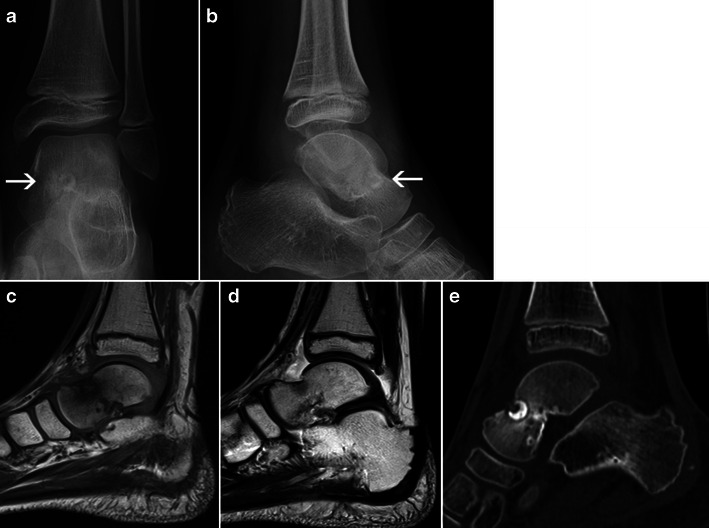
Osteoid osteoma of the left talar neck: Frontal (a) and lateral (b) X-rays of the left ankle: large round sclerotic fragment within an osteolytic cavity (*arrows*); no surrounding reactive osteosclerosis is identified. c Sagittal T1-weighted MRI: intense bone oedema surrounding the lesion. d Sagittal T2-weighted MRI: an intense inflammatory reaction is identified within the ankle joint. e CT, sagittal reconstruction: confirmation of the large nidus, entirely filled by the sclerotic fragment and with almost no surrounding osteosclerosis
